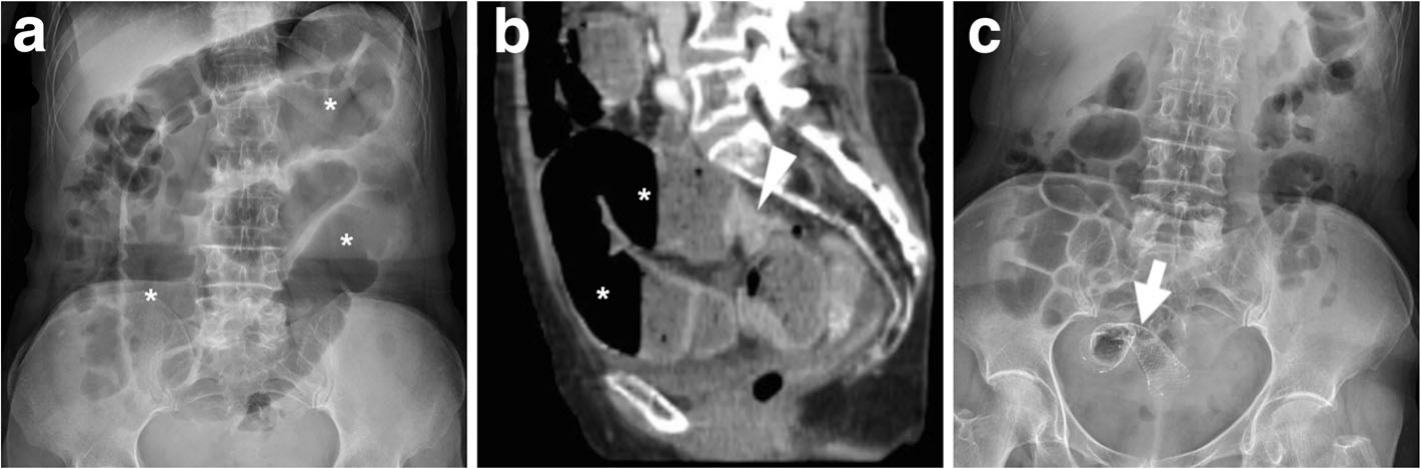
Effective relief of LBO during bridge-to-surgery stenting of CRC. Initial supine (a) radiograph shows marked gaseous dilatation of the large bowel (*) consistent with LBO. Sagittal post-contrast CT image (b) confirm air-fluid colonic dilatation (*) above a short stricturing sigmoid tumour (arrowheads). Forty-eight hours after endoscopic positioning of a SEMS (thick arrow), a plain radiograph (c) shows near-complete resolution of LBO. Three weeks later, the patient successfully underwent sigmoid resection with termino-terminal anastomosis
